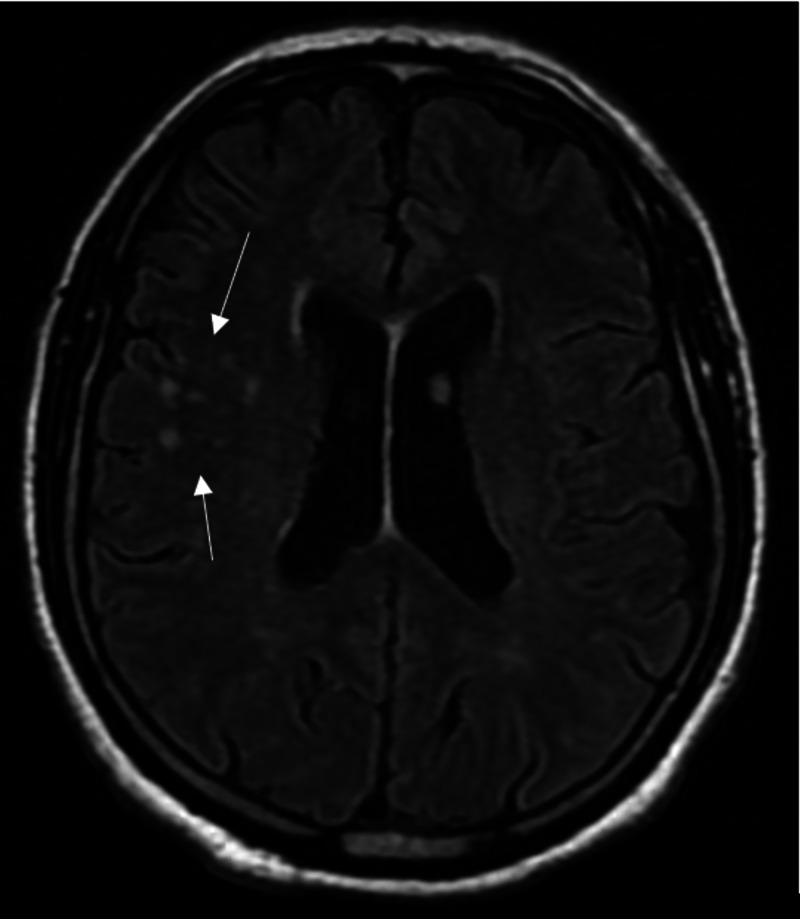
T2-FLAIR weighted magnetic resonance imaging (MRI) of the brain showing chronic cerebral demyelination (see arrows) FLAIR - fluid-attenuated inversion recovery
